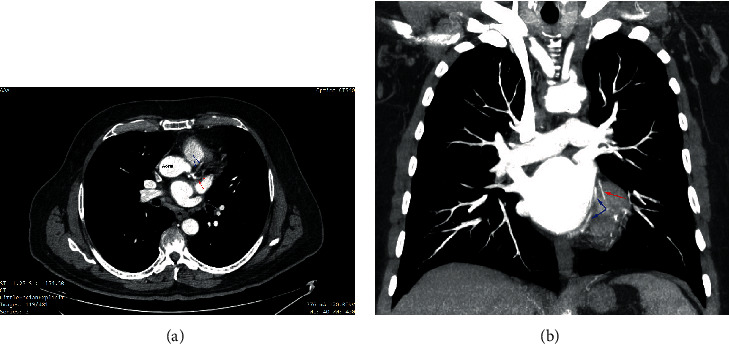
CT angiography of the aorta revealing a short LMCA (a) which then splits into two distinct branches (pointed with arrows of different color) descending toward the apex in the anterior interventricular groove. The short LAD is pointed with blue arrows and the long LAD with red arrows.
